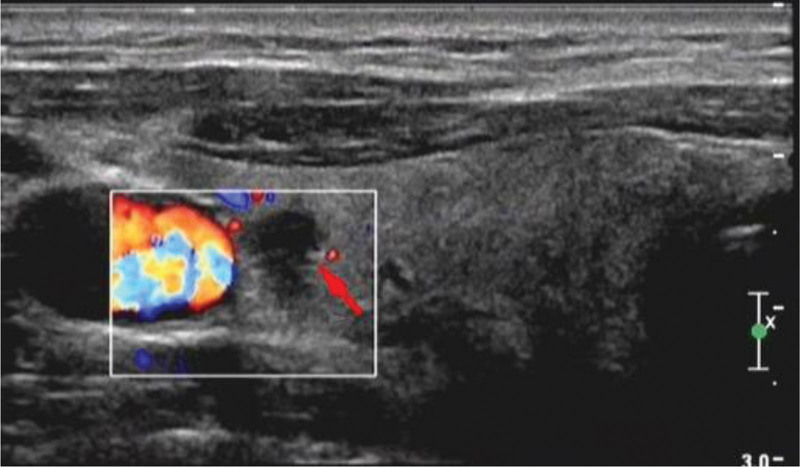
Color Doppler ultrasound of the right thyroid lobe shows a solid hypoechoic nodule (red arrow) in the upper pole with irregular margins, taller-than-wide shape, absence of hypoechoic halo, and scant intralesional vascularity. The lesion was classified as TI-RADS 5. TI-RADS 5 = thyroid imaging reporting and data system 5.
